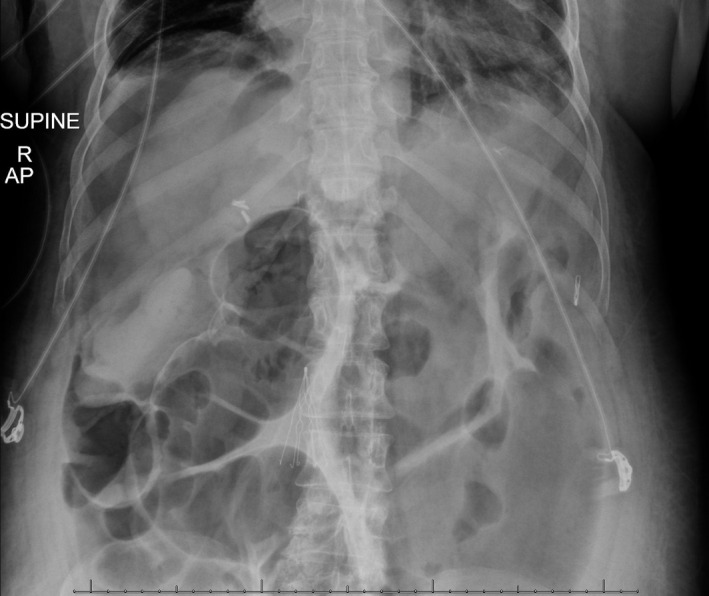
Abdominal radiograph revealing signs of abdominal perforation
